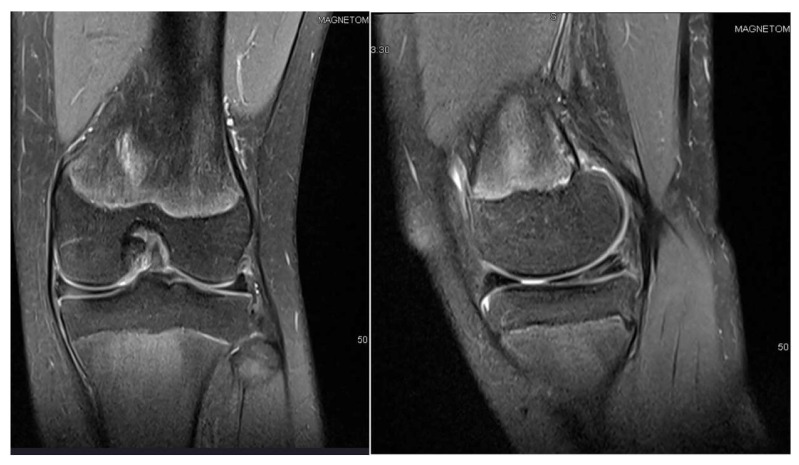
Patient S.C.S. (13 years old). Preoperative MRI of the left knee diagnosed with a medial discoid meniscus (left—coronal T2 view; right—sagittal T2 view). The coronal image demonstrated an abnormally enlarged and thickened medial meniscus extending across the tibial plateau, consistent with a complete discoid morphology. The sagittal view revealed a horizontal cleavage tear in the posterior horn.
